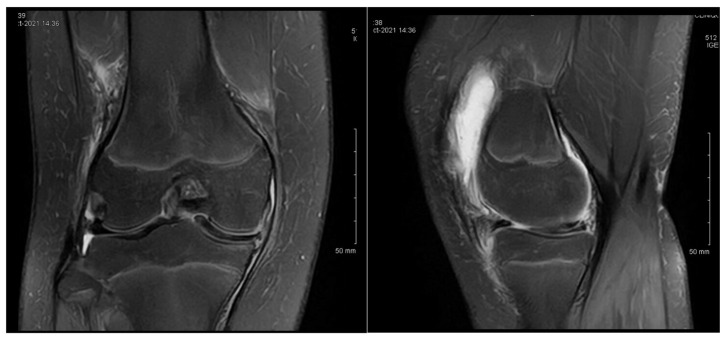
Patient P.E. (12 years old). MRI images of the right knee demonstrating features of a medial discoid meniscus (left—coronal view; right—sagittal view). The coronal T2-weighted image showed a thickened and abnormally widened medial meniscus extending across the medial tibial plateau, consistent with a discoid morphology. The sagittal view revealed increased signal intensity and an altered morphology in the posterior horn. Mild joint effusion was also present. An initial MRI was performed before the first surgery.
